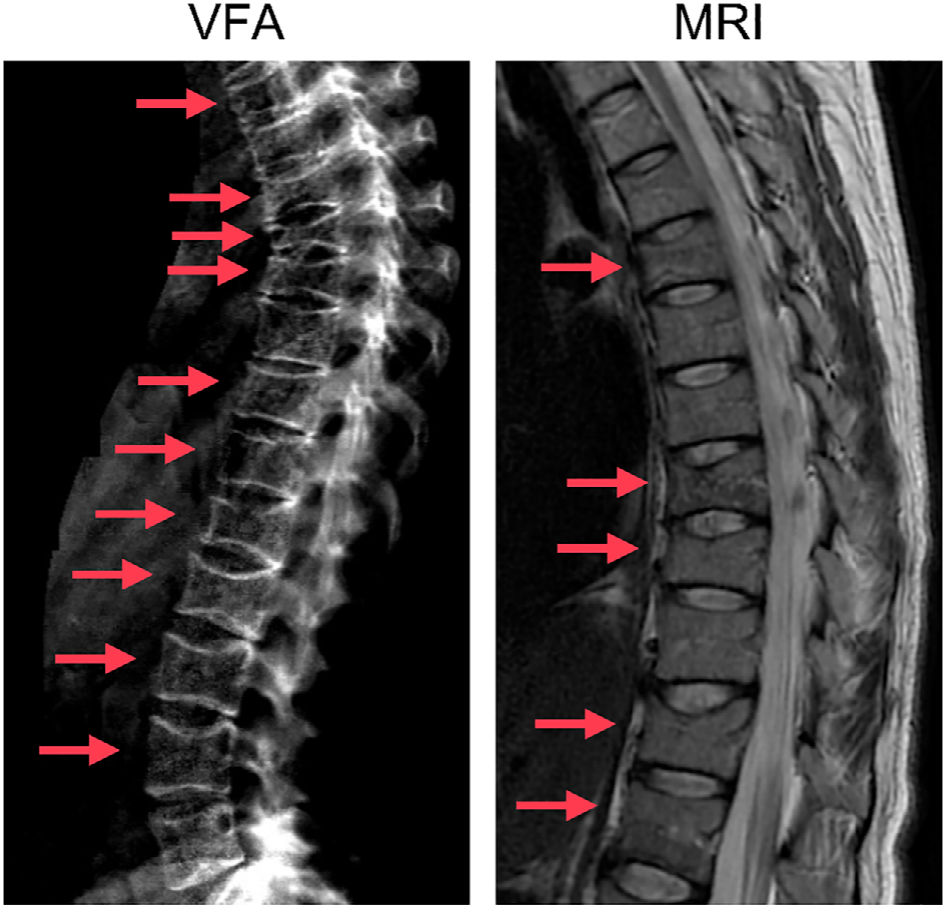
PLO manifestation. Representative vertebral fracture assessment (VFA, left panel) and MRI image of multiple vertebral fractures of two women (left: Individual 3, right: Individual 1) with a history of PLO.
